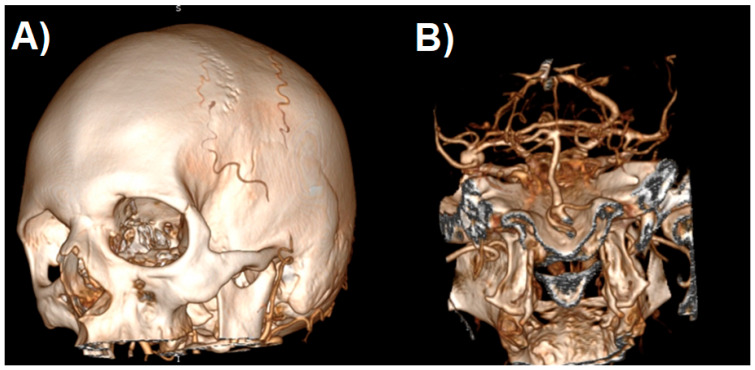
Shaded surface display volume rendering technique reconstruction of CT-angiography of patient with cerebral aneurysm: (A) raw reconstruction. (B) Circle of Willis after initial cropping.
